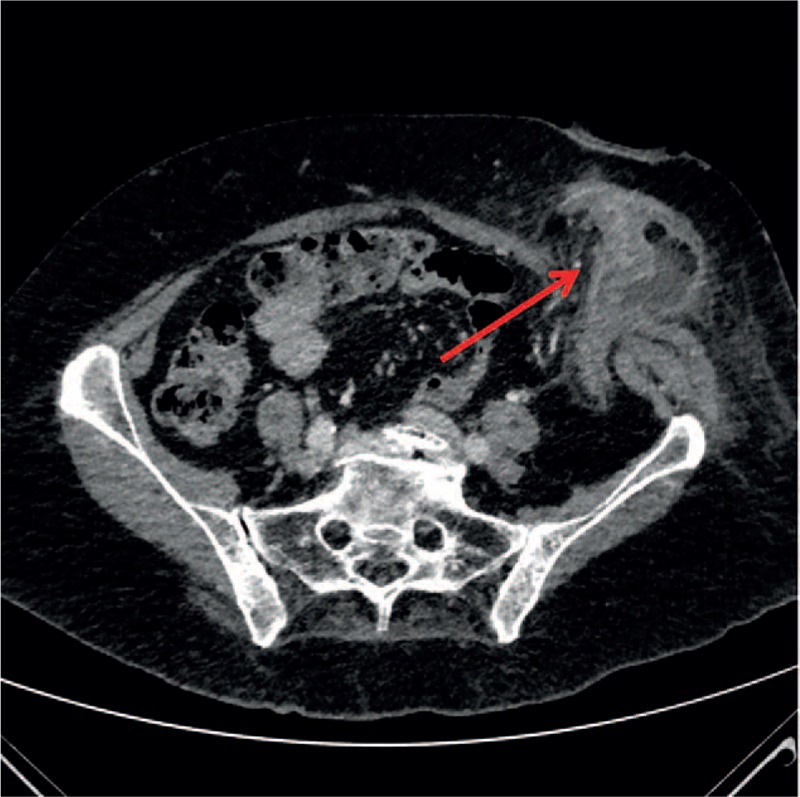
Computed tomography: acute noncomplicated diverticulitis of end-colostomy (red arrow).
